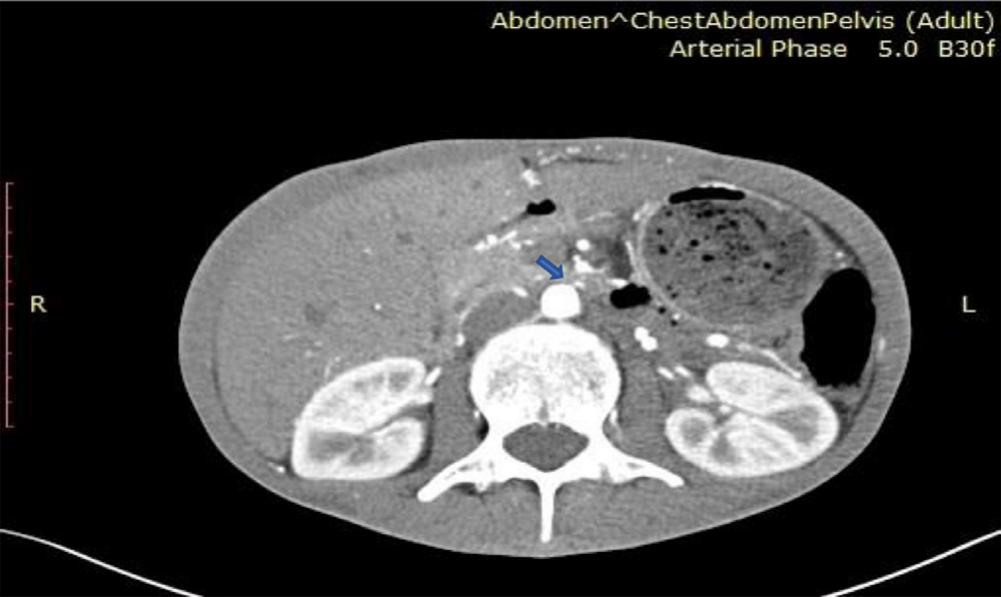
IMA stenosis on the axial view of CT Angiography with arterial phase.
